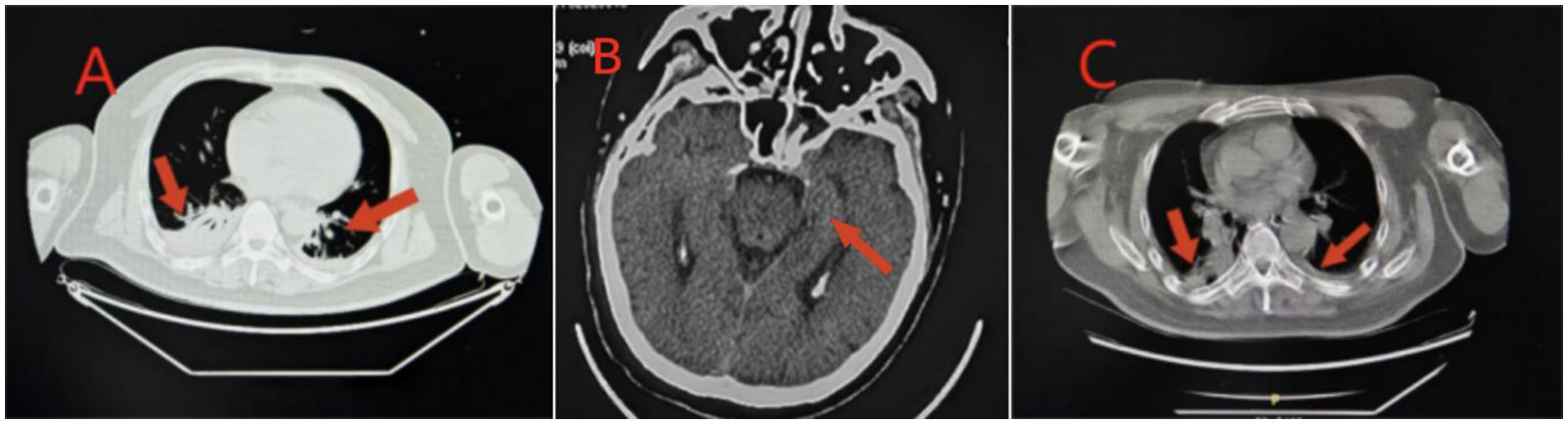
Imaging findings in Case 3: Panel (A) Lung CT shows inflammation in the lower lobes of both lungs, which is more prominent on the right side; Panel (B) Re-examination of brain CT shows ischemic degenerative foci in the bilateral basal ganglia and corona radiata; Panel (C) Lung CT shows inflammation in both lungs, and inflammation in the upper lobe of the right lung.
